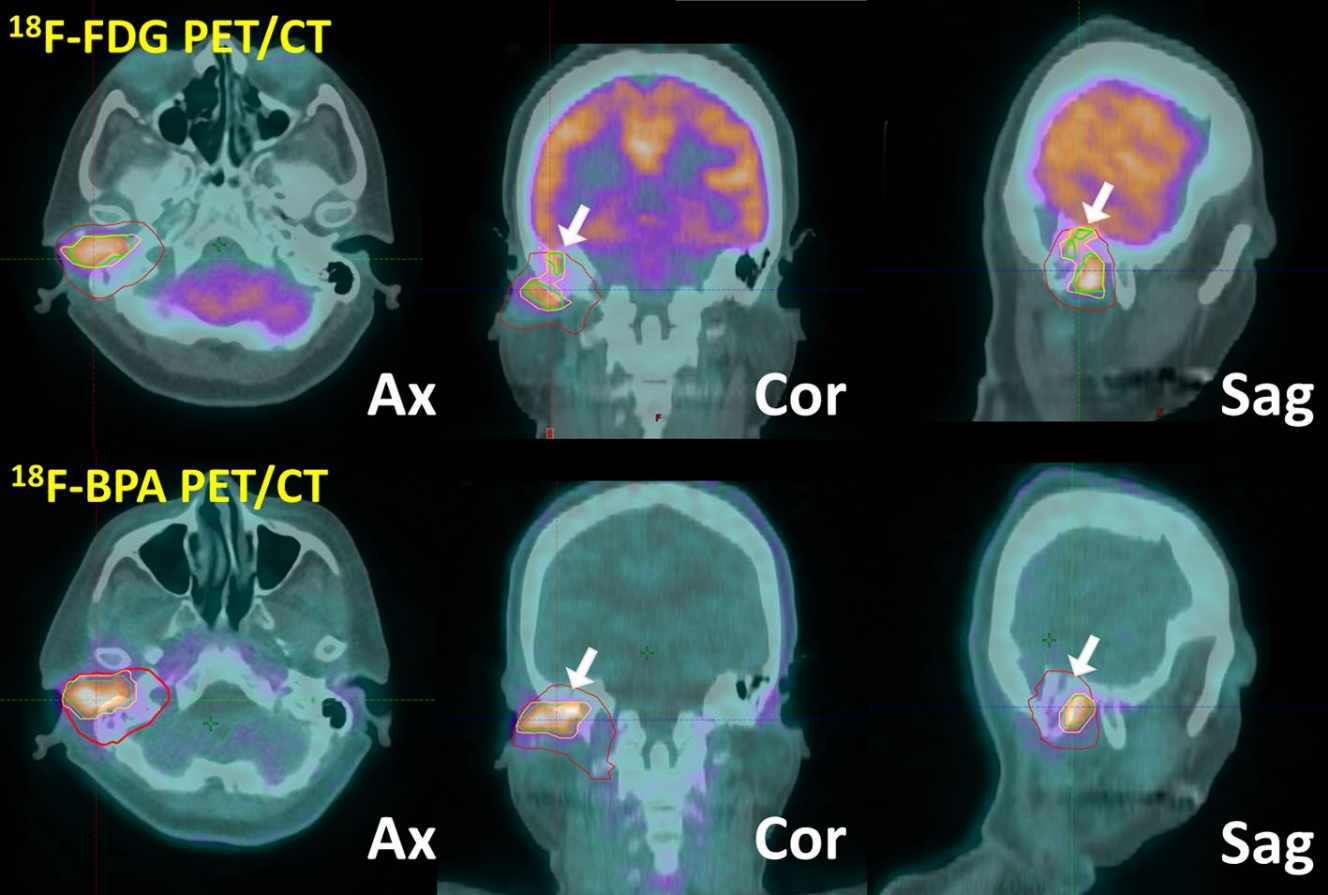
A 50-year-old woman was diagnosed with squamous cell carcinoma in the right external ear. The top and bottom images show fused images of ^18^F-FDG PET/CT and those of ^18^F-BPA PET/CT, respectively. The solid line with red, yellow, and green indicates the contour of GTV, MTV40, and MTV50, respectively. The discrepancy (white arrows) between two tracers is observed in coronal and sagittal planes due to physiological brain accumulation or inflammation (mastoiditis or external otitis)
